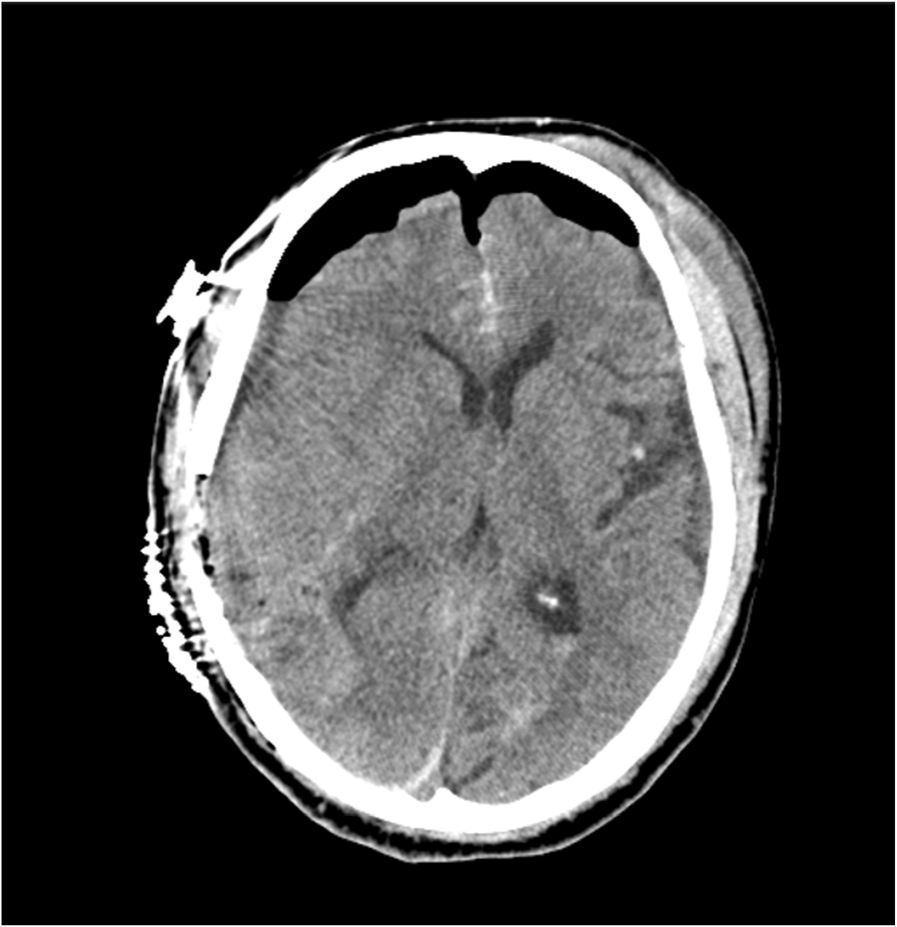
Head CT after surgery showed the removal of the hematoma
